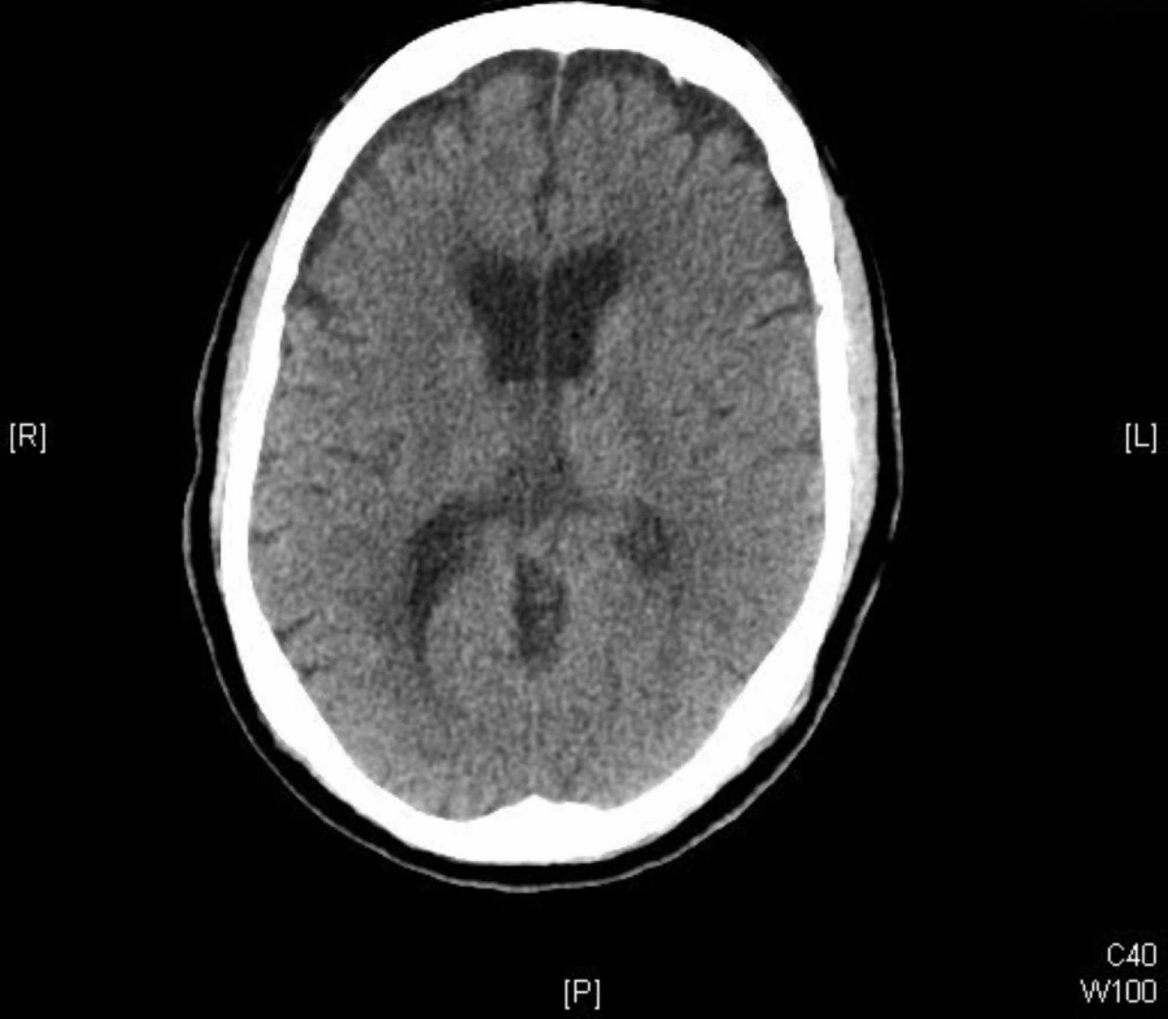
Noncontrast brain CT demonstrates resolution of the pneumocephalus.
